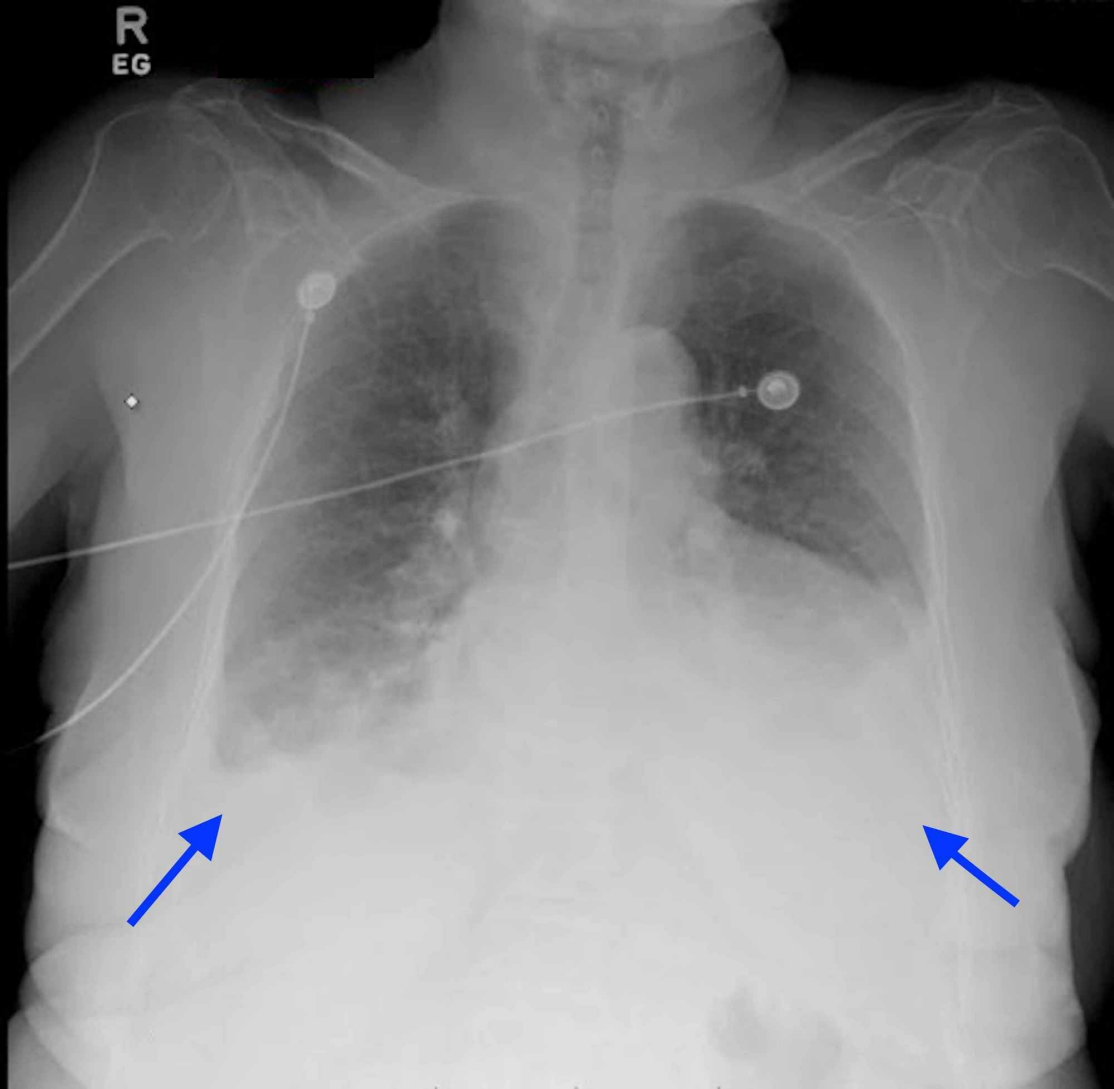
Chest X-ray shows bilateral pleural effusions (blue arrows) and cardiomegaly.
